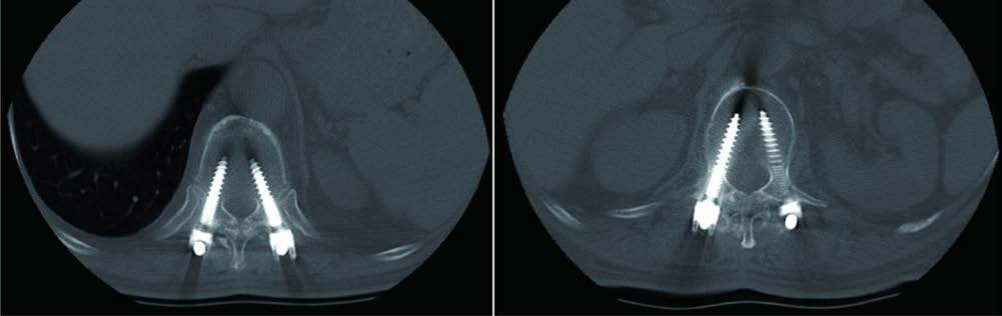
Postoperative X-ray scans show that screw position and titanium rod length are suitable (Grade 0) (on the left side is T11, on the right side is L1).
